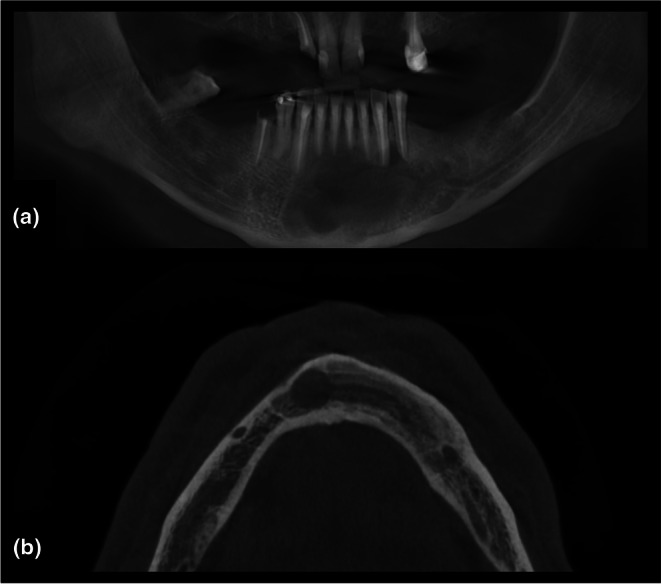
Cropped cone‐beam computed tomography images revealing a radiolucent lesion in the anterior mandible: (a) 15‐mm‐thick panoramic reconstruction; (b) axial reconstruction. Note the absence of buccolingual expansion, which is more clearly appreciable in the axial view, underscoring its added diagnostic value.
